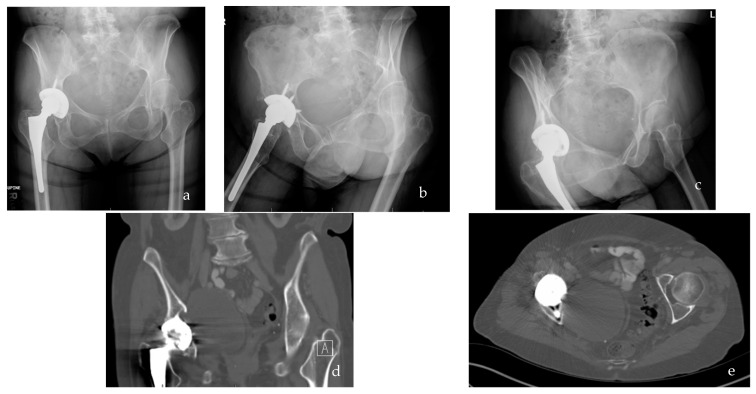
(a–c) AP pelvis and Judet radiograph demonstrating late-stage osteolysis of the right total hip arthroplasty as evidenced by lucency around the implant-bone interface of the acetabular cup. (d,e) Coronal and axial computed tomography (CT) demonstrating late-stage osteolysis of the rigt total hip arthroplasty as evidenced by lucency throughout acetabular cup periphery, and ischium.
